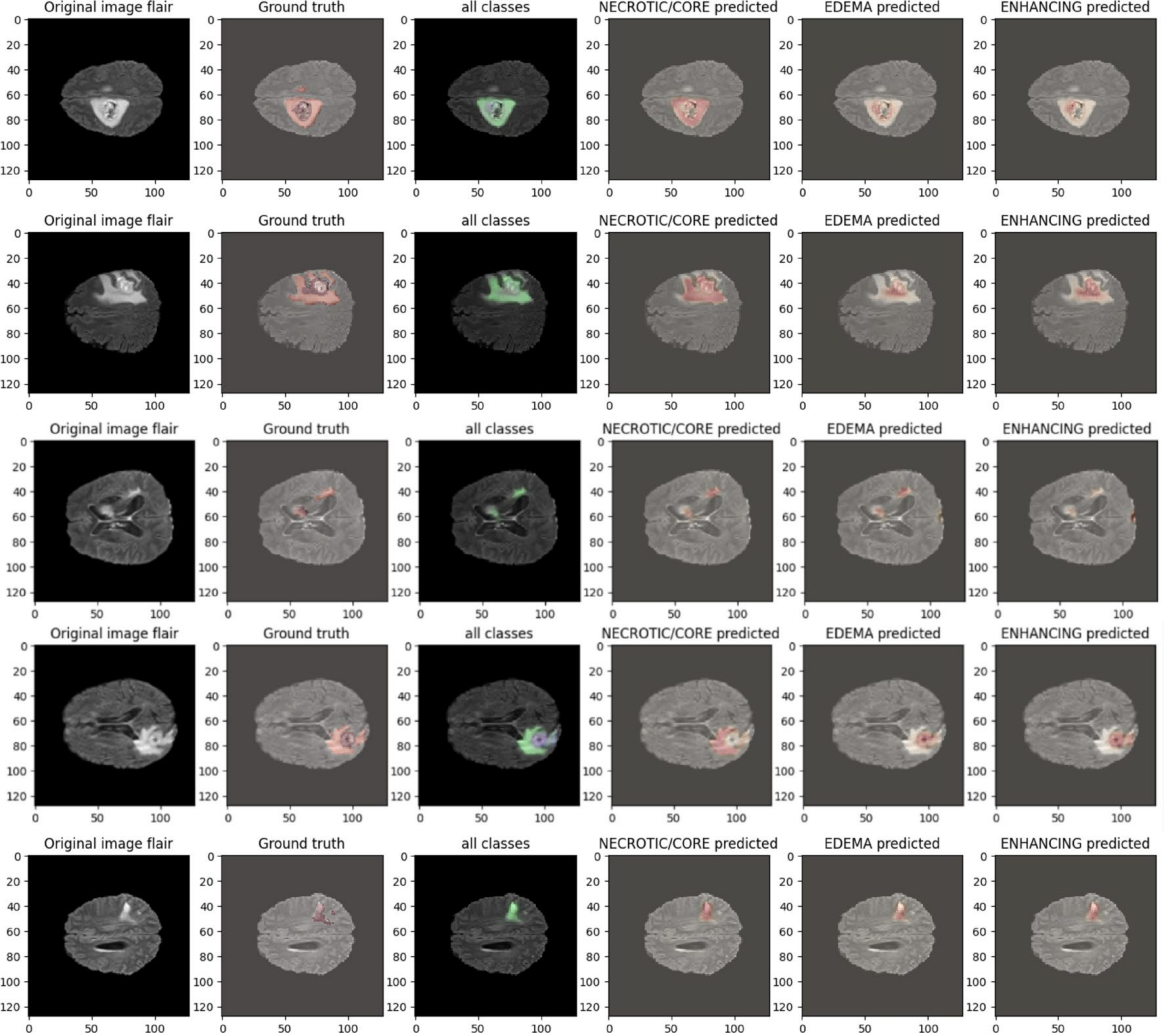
After training the model, the following visualization showcases the qualitative results of the IC-Net model on BraTS2020 MRI sequences.
